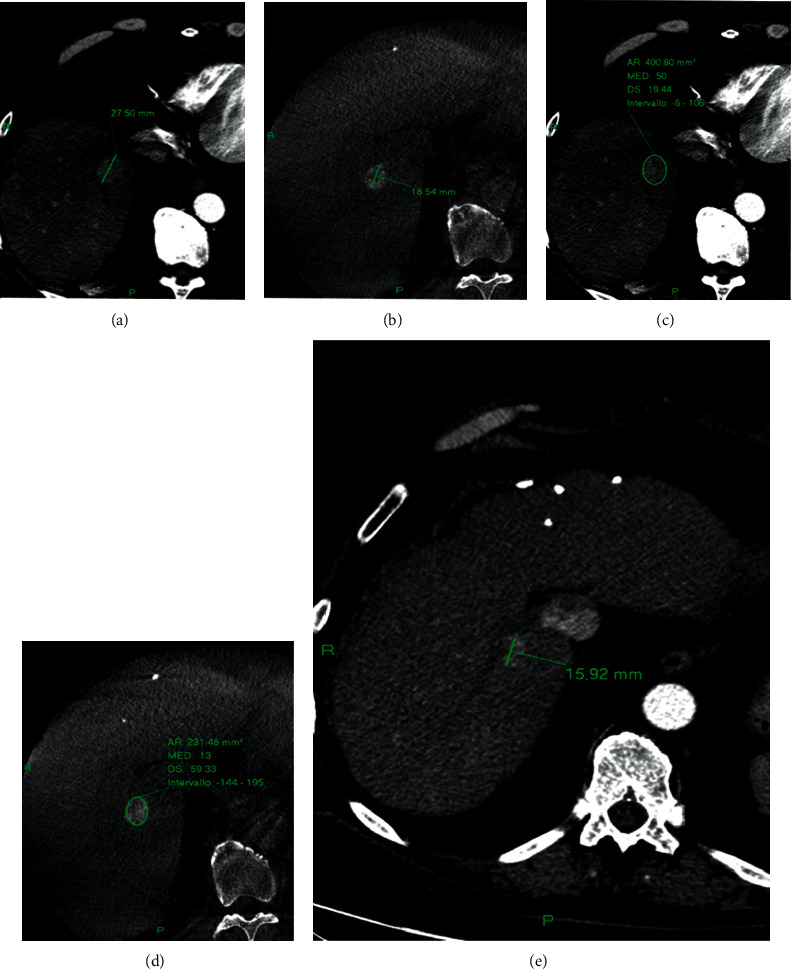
Diameter and density of an HCC nodule on the preprocedural CT (a, c) and on the CBCT (b, d). Contrast enhancement CT at 1 month from the procedure shows not complete response to the DSM-TACE (e).
